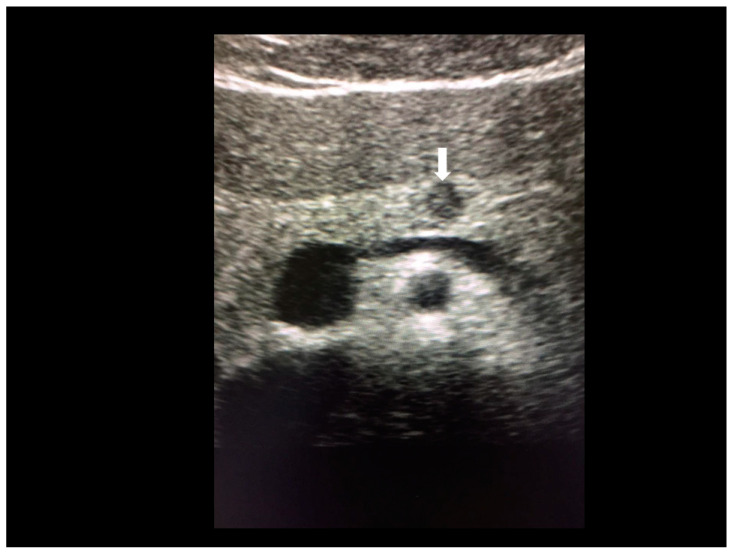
Endometriosis of pancreas (arrow) confirmed at MRI.
